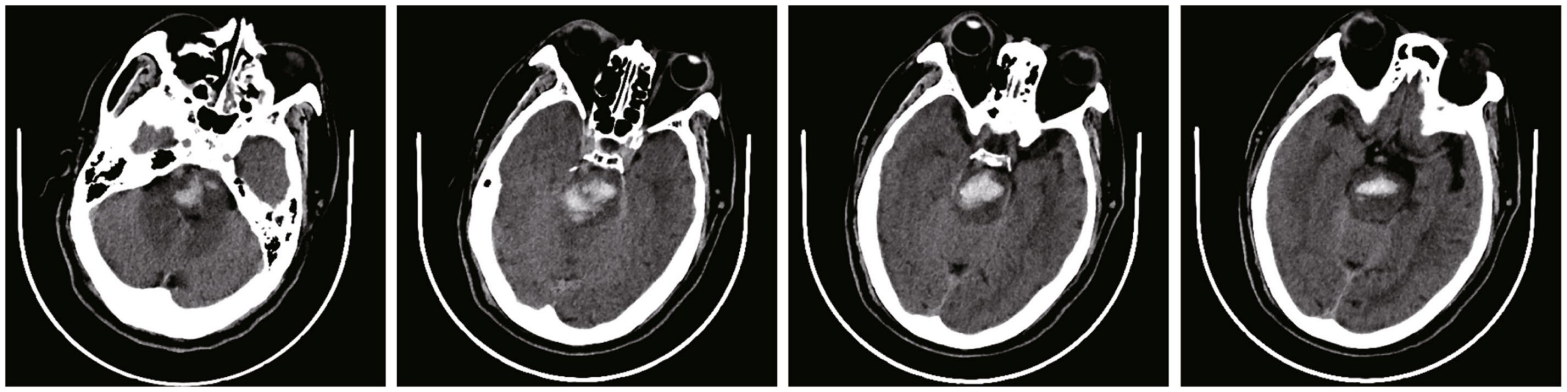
Pre-operative CT scans of a 61 year-old male patients at admission, with a pontine hemorrhage of 7.9 mL (The images in this manuscript were all from the same patient).
